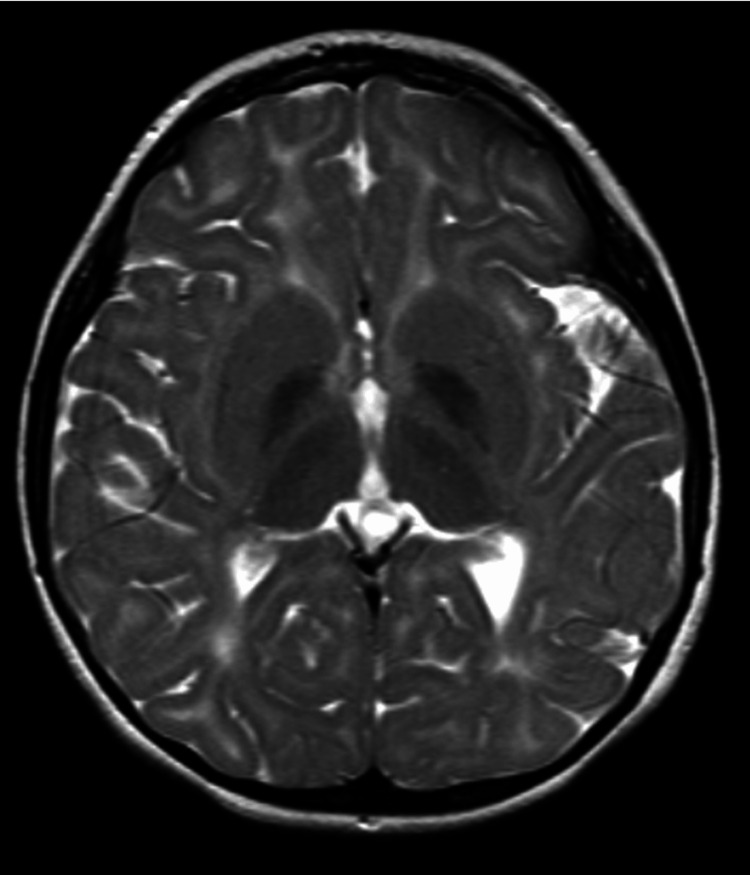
Axial T2 Weighted Imaging Axial T2-weighted image obtained at the level of basal ganglia demonstrated subtle T2 prolongation within the putamina.
